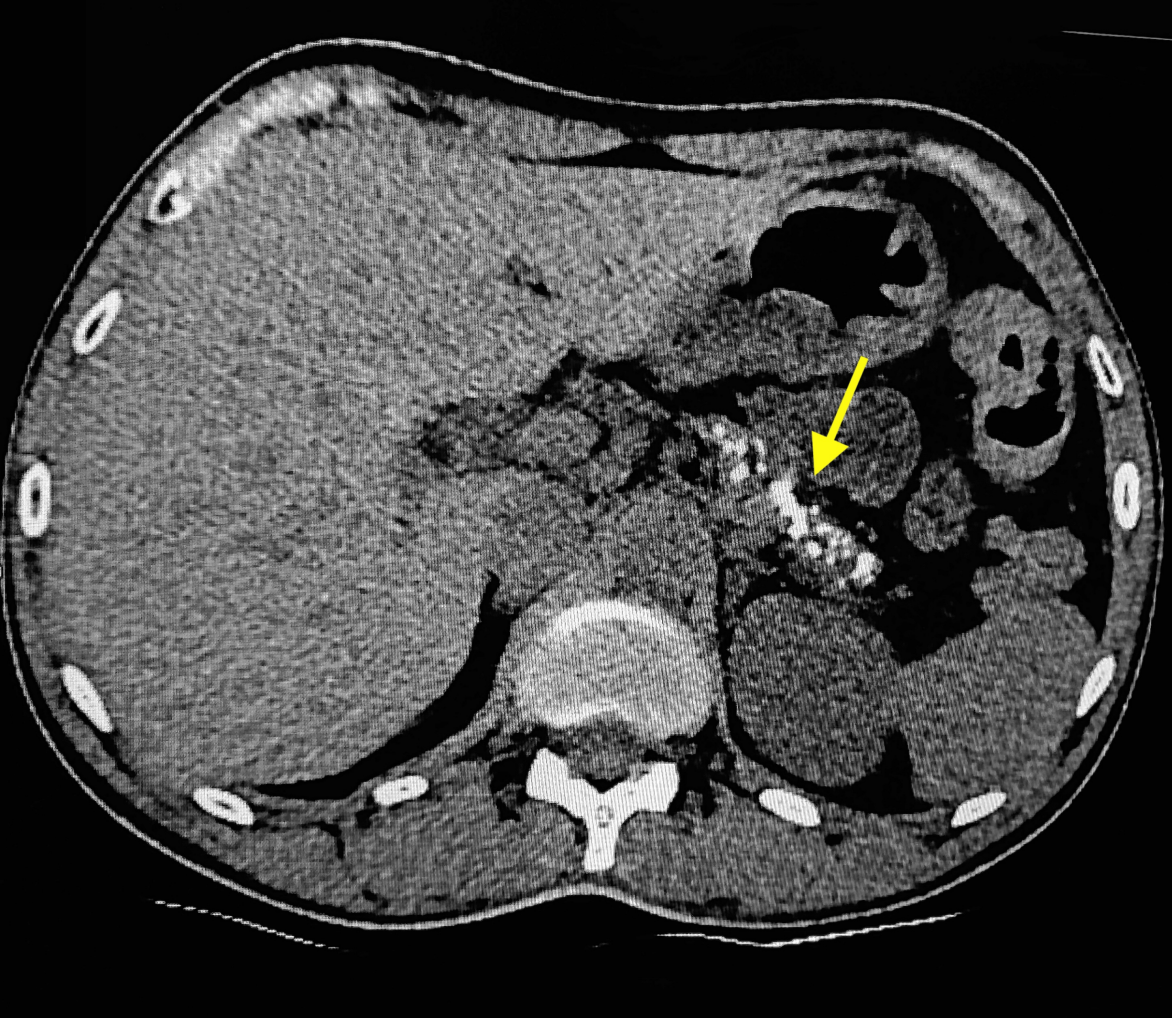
Non-contrast CT of the abdomen showing chronic calcific pancreatitis (yellow arrow). CT: Computed tomography
